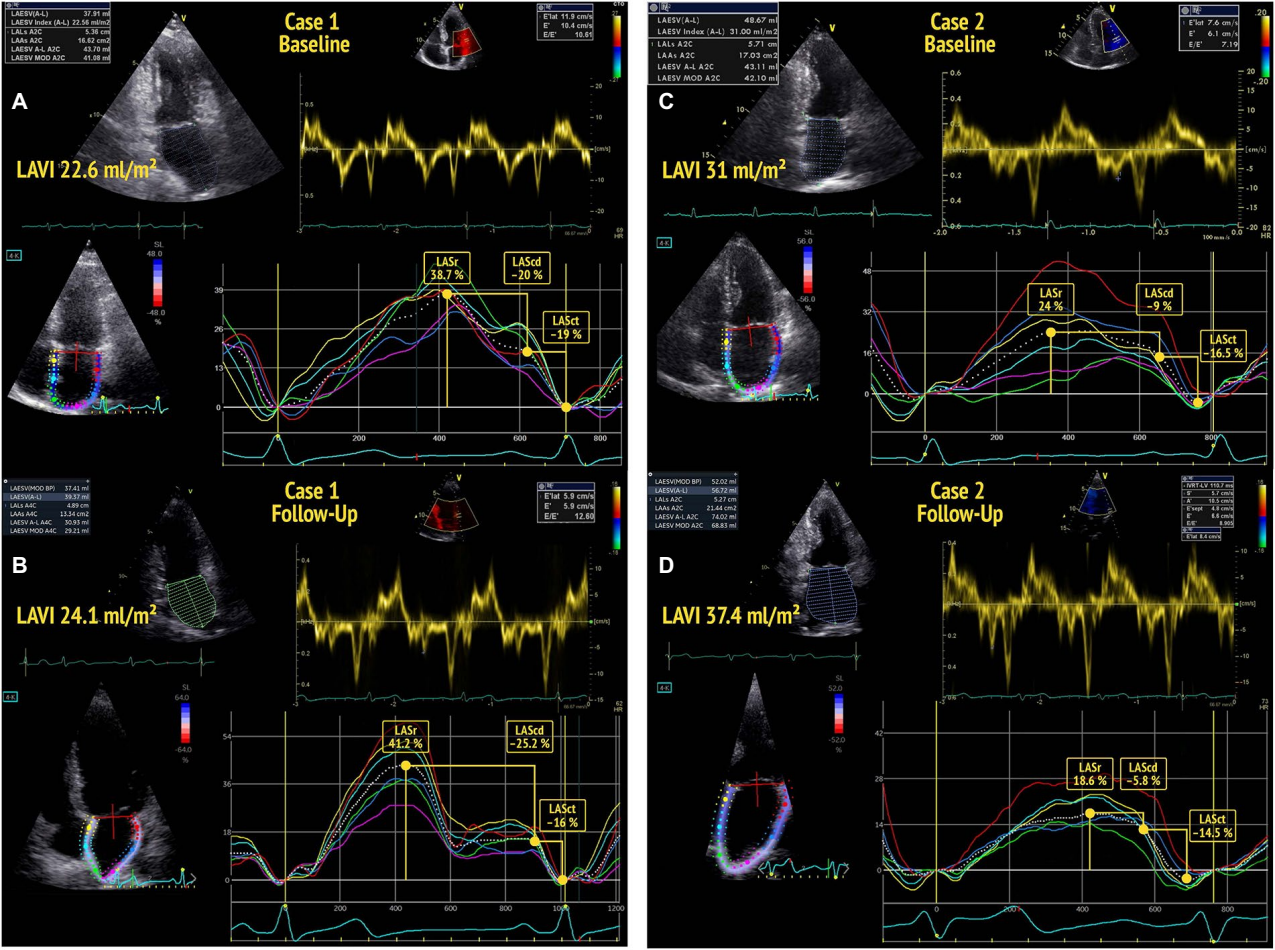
Baseline echocardiography of two participants (A,C) showing normal left atrial volume index (LAVI) (<34 ml/m^2^) and normal diastolic function according to the 2016 ASE guidelines. The participant in A shows a left atrial reservoir strain (LASr) >34% at baseline and no deterioration of diastolic function by the time of follow-up (B). The participant in C presents with a low baseline LASr and shows a decline of diastolic function (DD0 to DD1) by the time of follow-up echocardiography (D).
